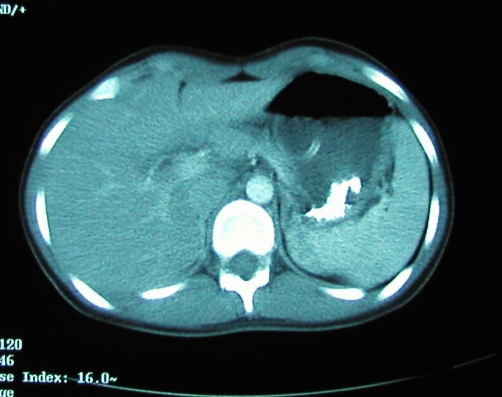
CT confirming hepatitis.
